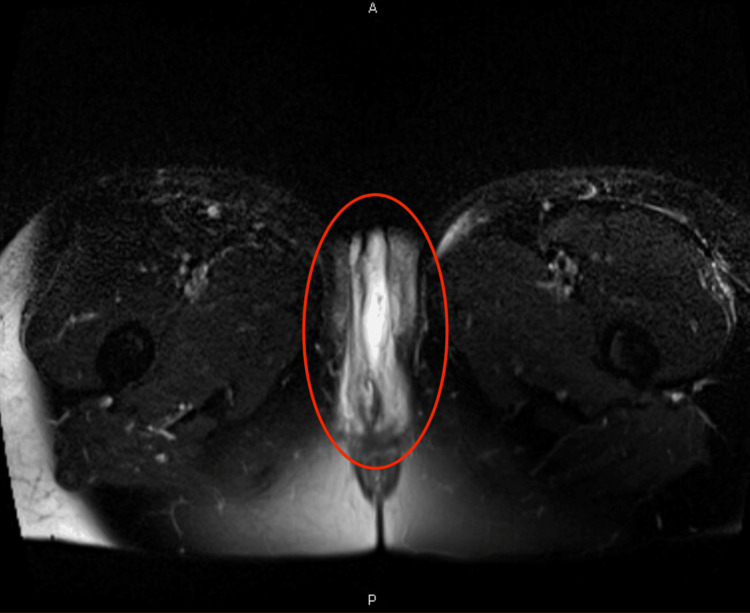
MRI of the pelvis without contrast Axial view showing bilateral labial edema.
